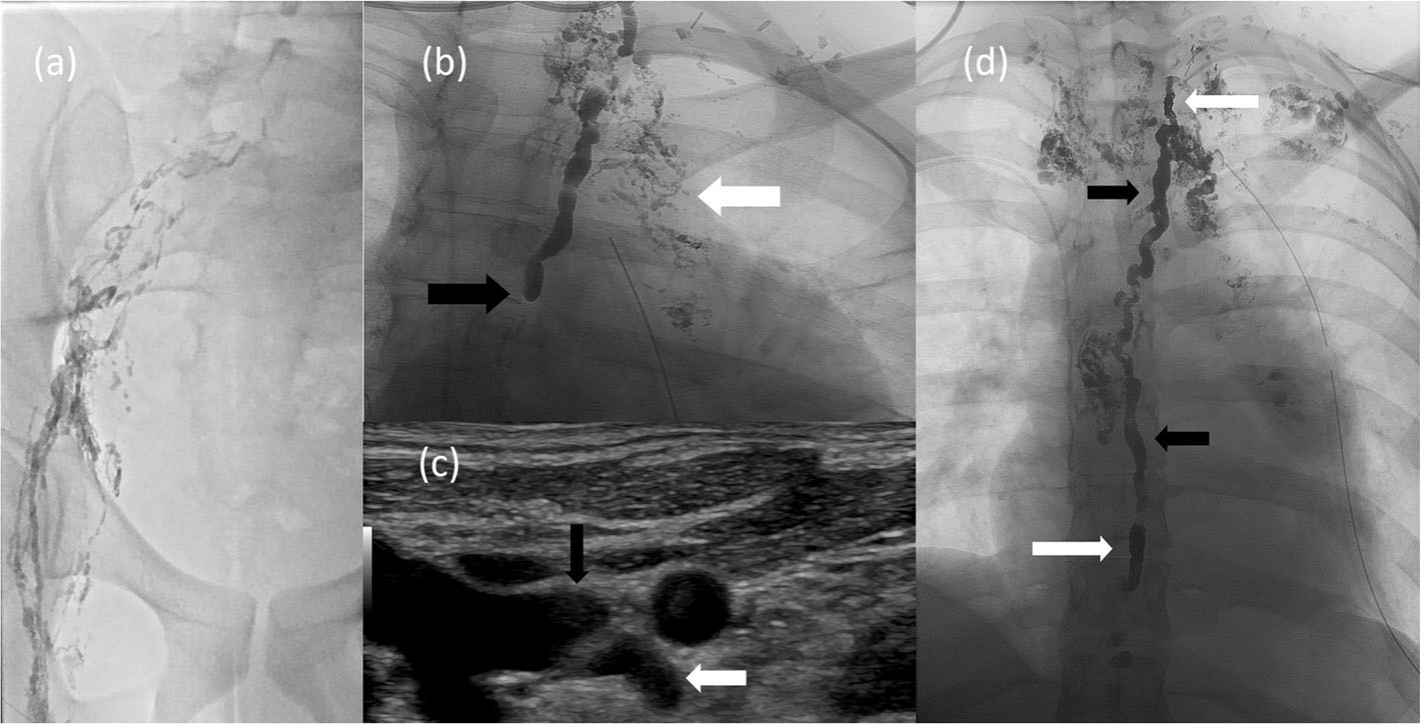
Intra-procedural findings. Lymphangiogram after ethiodol injection into a groin lymph node (a) revealed abnormal dilated and tortuous lymphatic channels in the right iliac chain. Fluoroscopic image (b) of the upper chest demonstrated a partially opacified thoracic duct terminating abruptly at the inferior margin (black arrow) and multiple abnormal, irregular lymphatic channels (white arrow) in the mediastinum corresponding to the CT image in Fig. 1. The abrupt termination was likely due to a valve. This was crossed without much difficulty with a microsystem. Grayscale ultrasound image (c) of the thoracic duct (white arrow) draining into the left subclavian vein (black arrow) at the venous angle. Intra-procedural fluoroscopic image (d) of the thoracic duct demonstrating embolization coils (white arrows) at the inferior and superior aspects of the duct. The intervening segment of thoracic duct was embolized with radiopaque NBCA (black arrows). A left chest tube and right PICC are present
